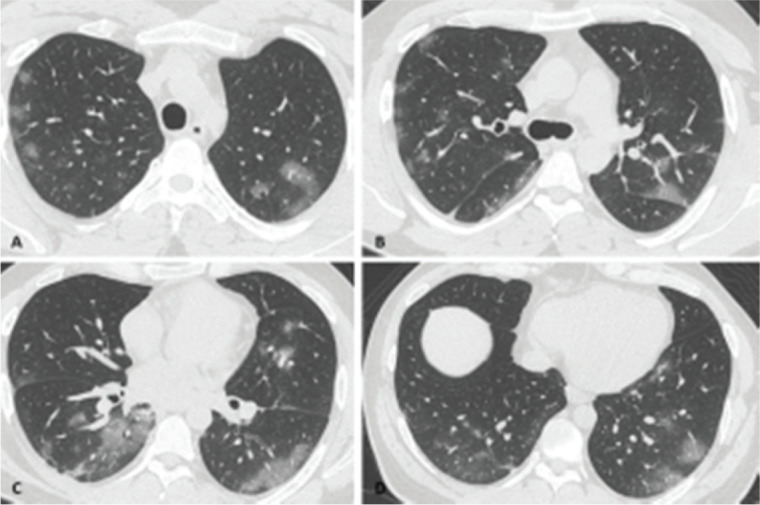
Typical findings of COVID-19. Axial CT images show multifocal GGOs of rounded morphology with areas of consolidation.
